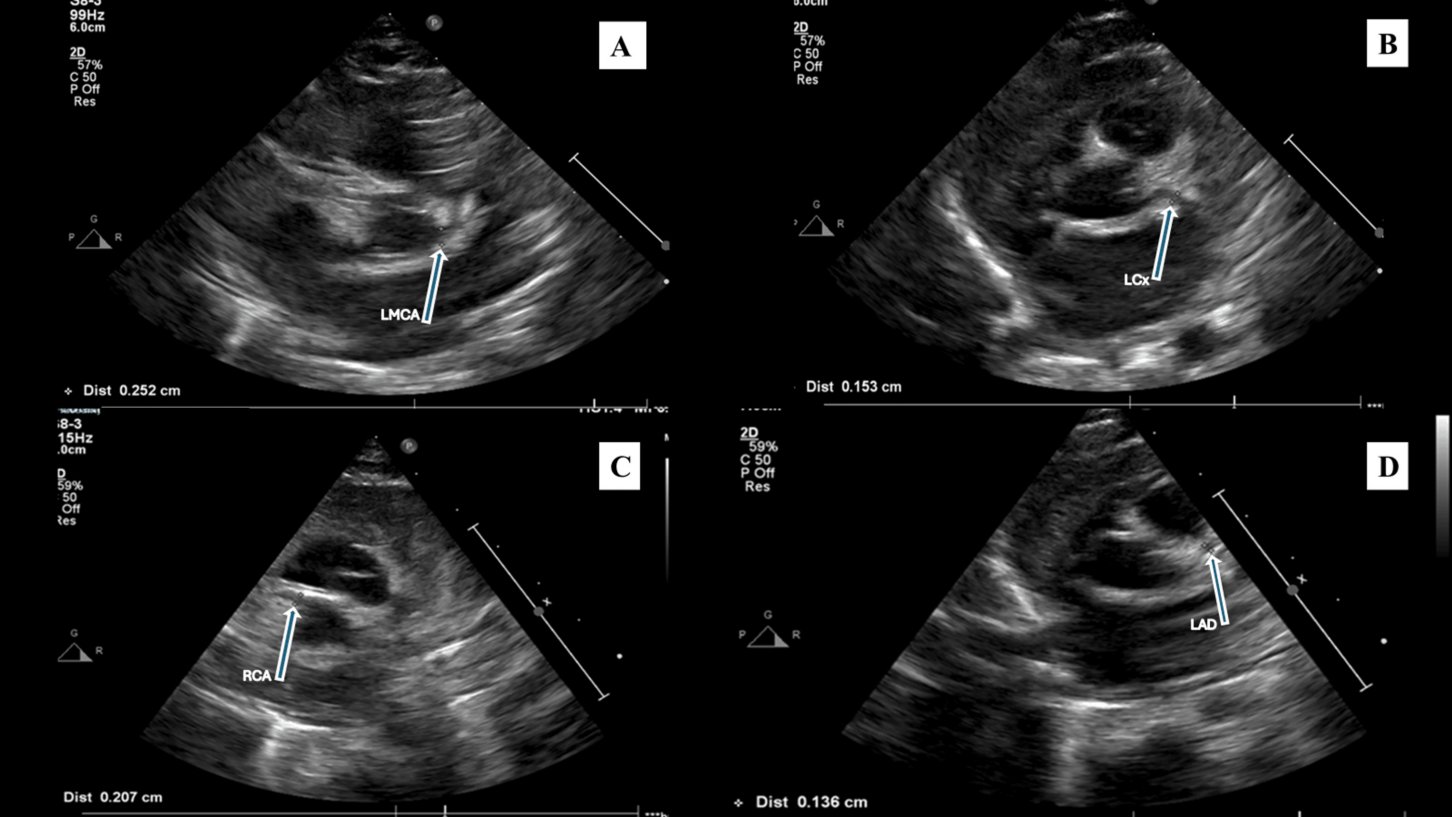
Follow-up transthoracic echocardiography demonstrating coronary artery dimensions after treatment Follow-up images showing the coronary arteries: (A) left main coronary artery, (B) left circumflex artery, (C) right coronary artery, and (D) left anterior descending artery. Arrows indicate the corresponding coronary artery segment, and the on-screen calipers (star-like markers) denote the measured coronary artery diameter
